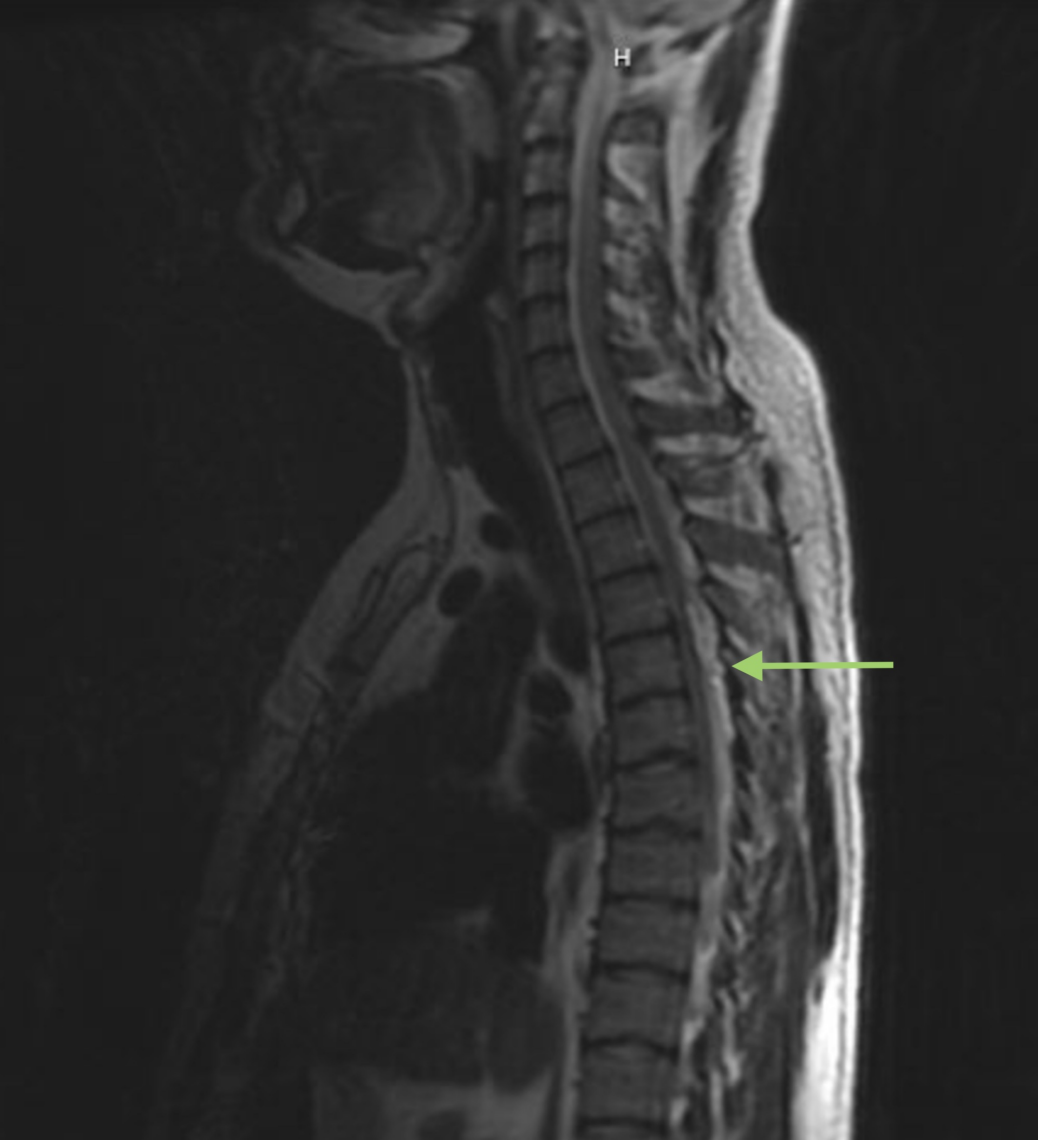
Sagittal MRI lumbar spine scout. Scout imaging revealed a hyperintense extradural lesion at the T4-T5 level indicated by the arrow.
